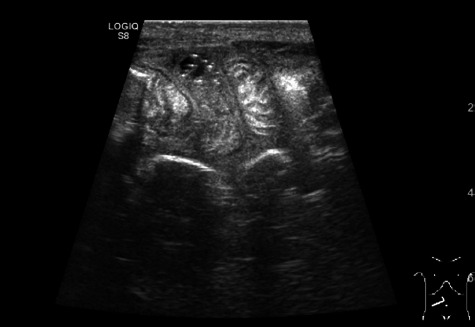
Ultrasonography on fourth day of admission showing oedemic pneumatized bowel
